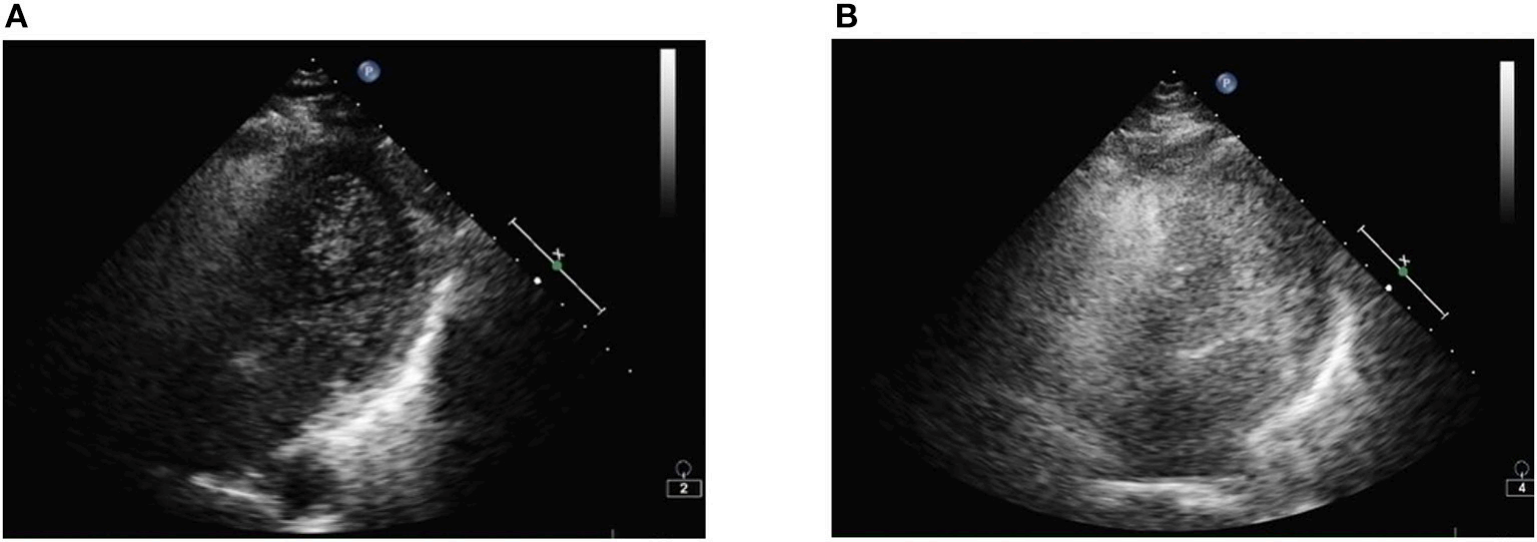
(A) After injection of agitated saline, microbubbles are visible in the left ventricle by transthoracic echocardiography. (B) After the Valsalva maneuver, a large volume of microbubbles is filling the left ventricular and subsequently the left ventricular.
